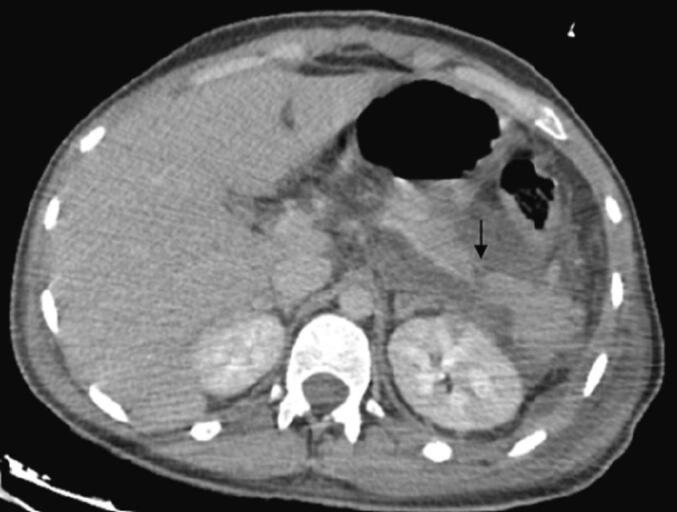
Axial section CT scan on day 3 of the trauma showing a transection of the pancreatic body.
